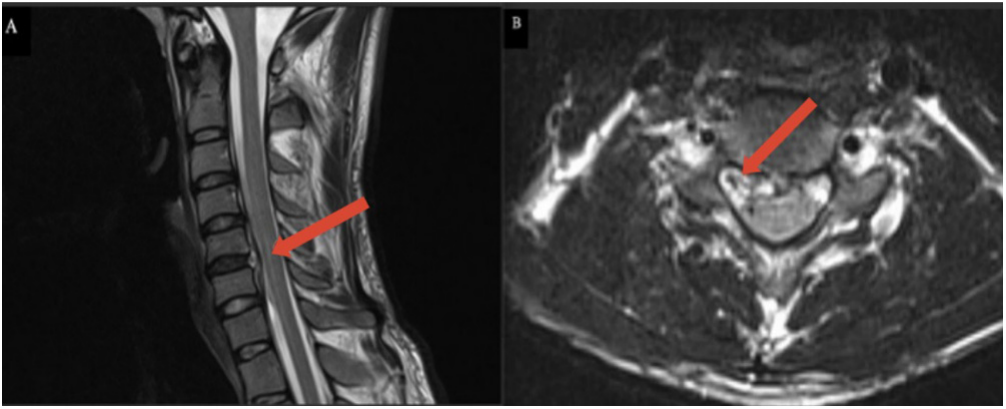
Sagittal (A) and Axial (B) views of T2-weighted image of cervical MRI showing small anterior extradural collection at C5/C6 levels extending more to the right side with mild mass effect.
